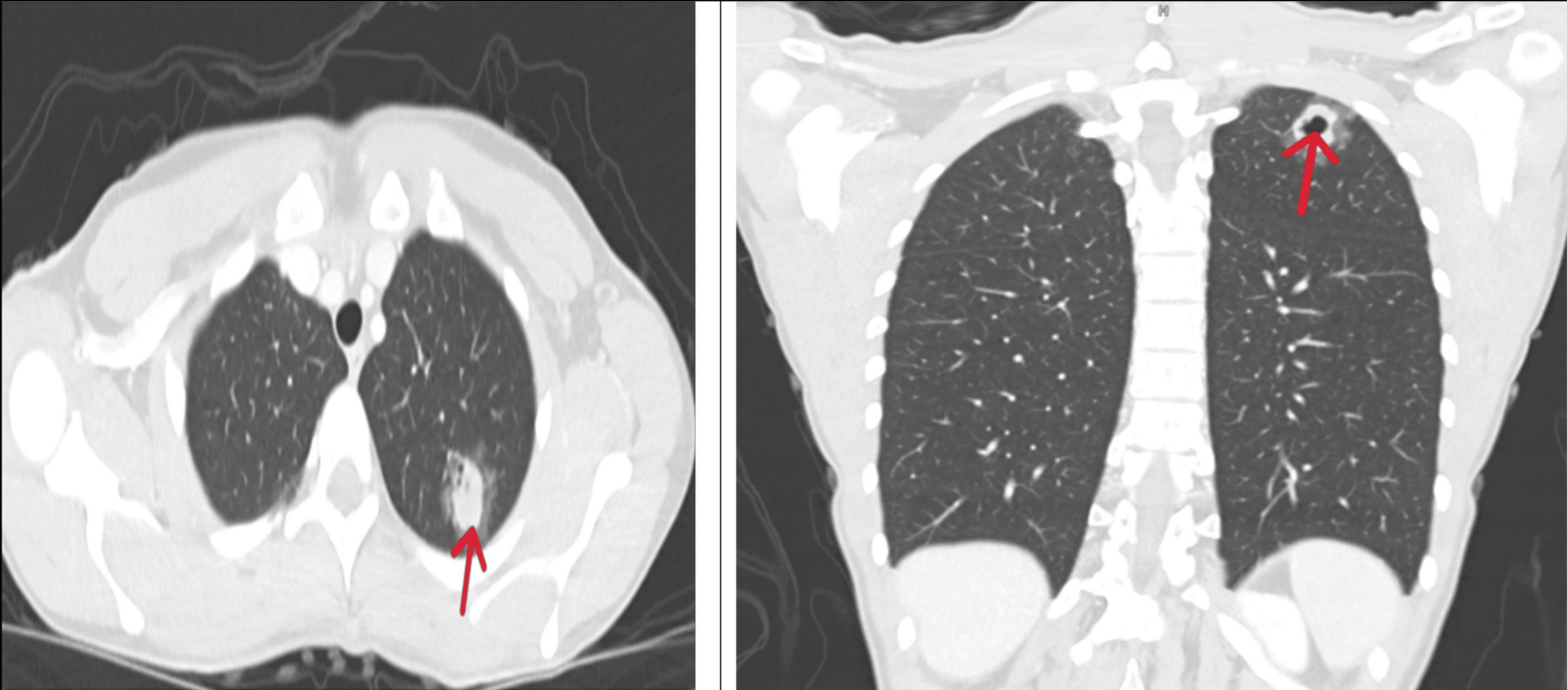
CT scan showing the left apical mass: axial and coronal views
